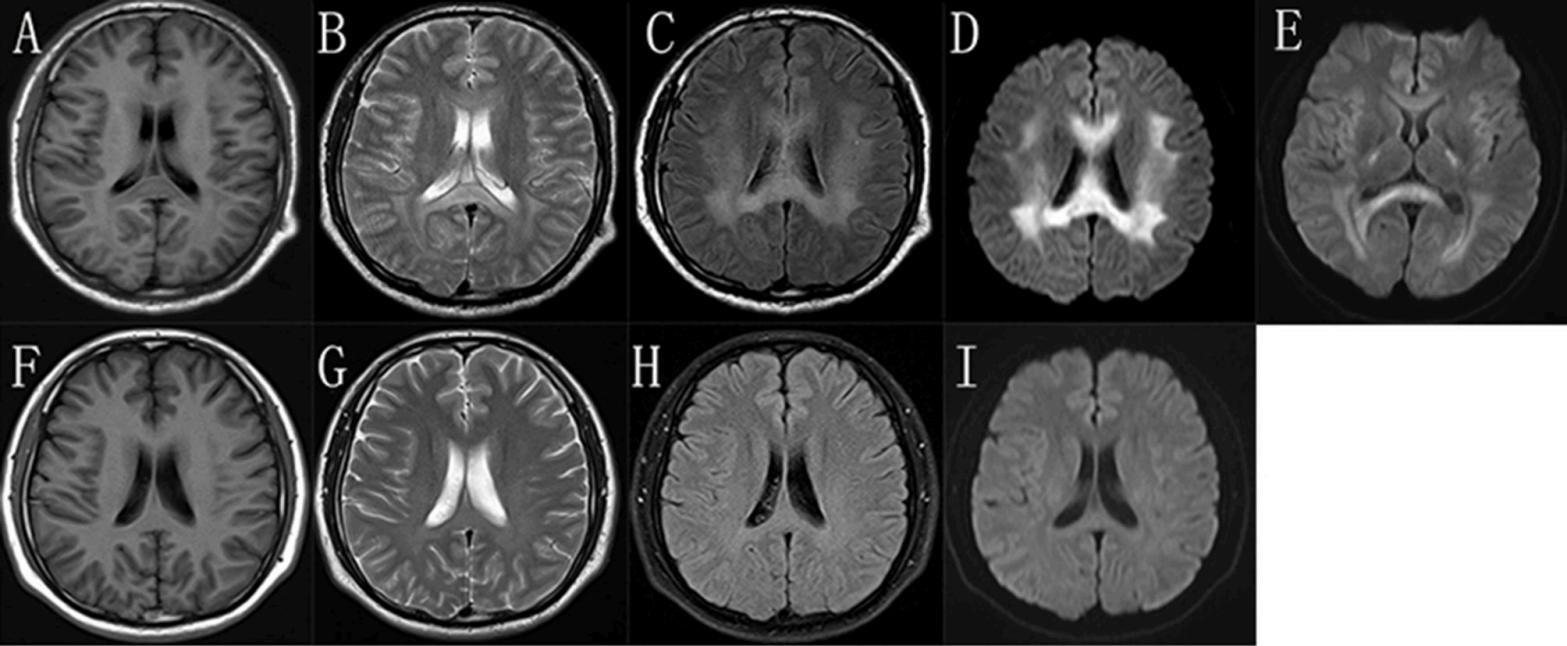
The brain MRI images of the proband. In the “stroke-like” episode, brain MRI showed slightly hypointensity in T1-weighted imaging (A) and slightly hyperintensity in T2-weighted imaging (B) in the bilateral deep white matter and the splenium of the corpus callosum. But there was obviously bilaterally symmetric hyperintensity in T2 FLAIR images and reduced diffusion in the same region (C,D). MRI after 8 days showed improvement of the abnormal diffusion signal in white matter (E). After 5 months, the images of T1-weighted (F), T2-weighted (G), T2 FLAIR (H), and DWI (I) of his brain were almost normal.
